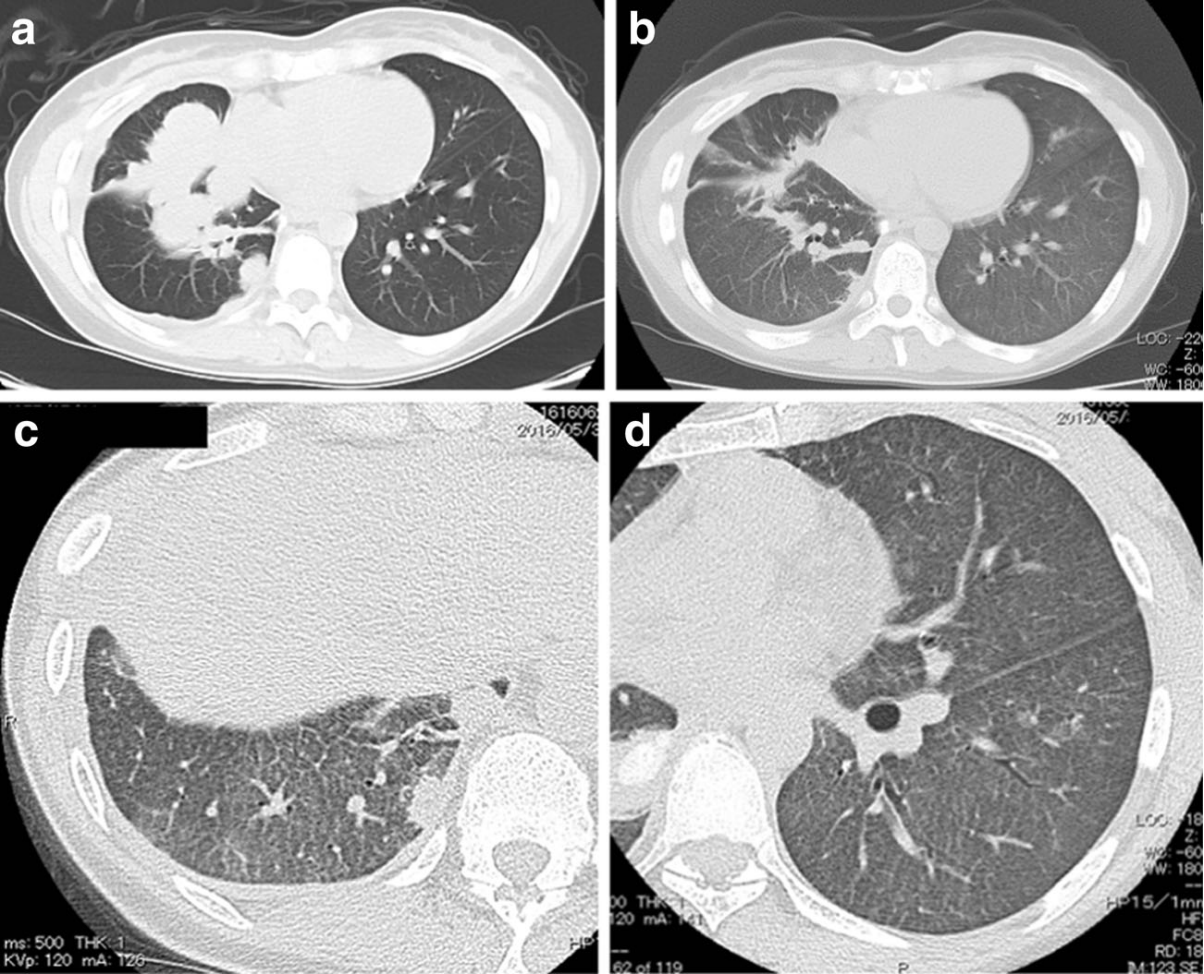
Chest CT findings before and after treatment. a Solid tumors with intrapulmonary metastasis and no evidence of preexisting interstitial pneumonia before treatment with osimertinib. b Remarkable shrinkage of tumors after treatment with osimertinib. c, d Diffuse, faint, ground-glass opacities lacking consolidation and traction bronchiectasis in both lungs after treatment with osimertinib
